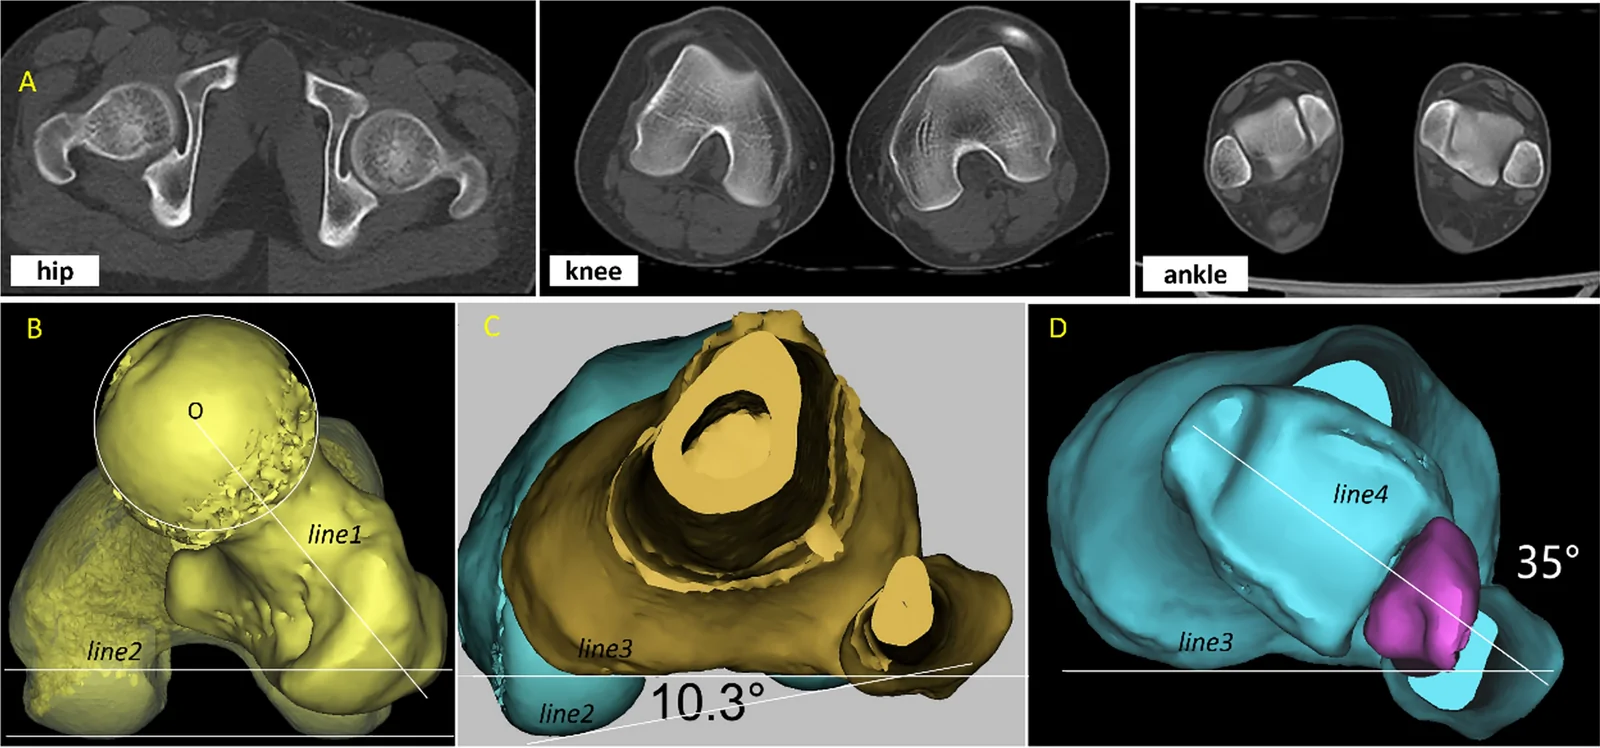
Standard CT scan of the hip-knee-ankle and measurements of (A) lower limb torsion parameters, (B) femoral anteversion, (C) knee torsion, and (D) tibial torsion
